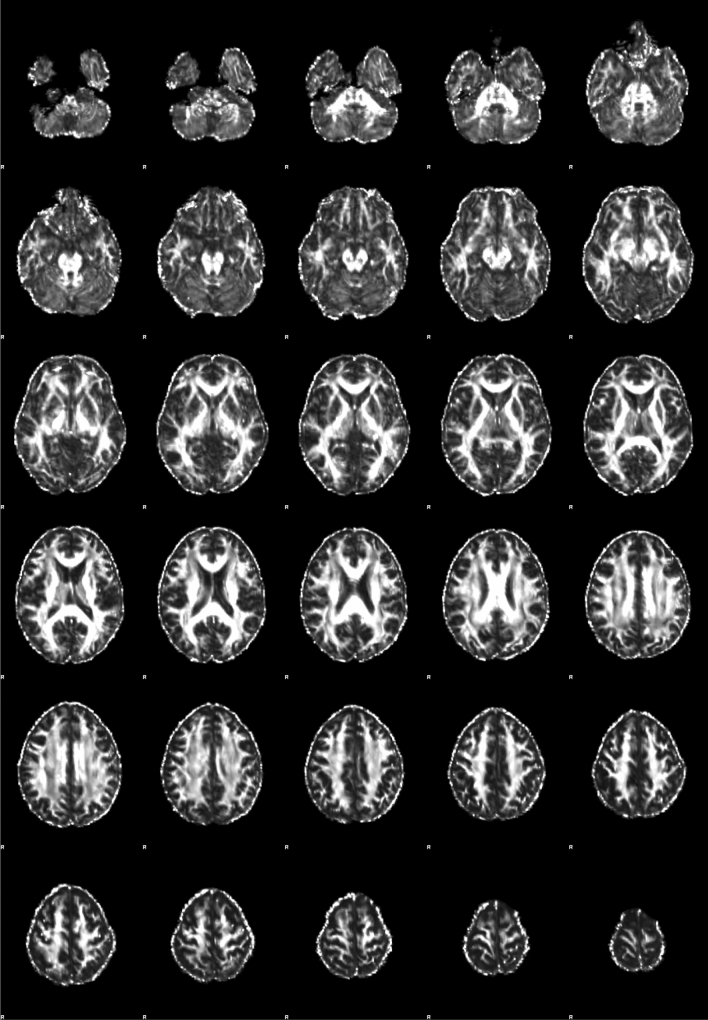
MRI slices of subjects with the smallest FA-BHQ.
